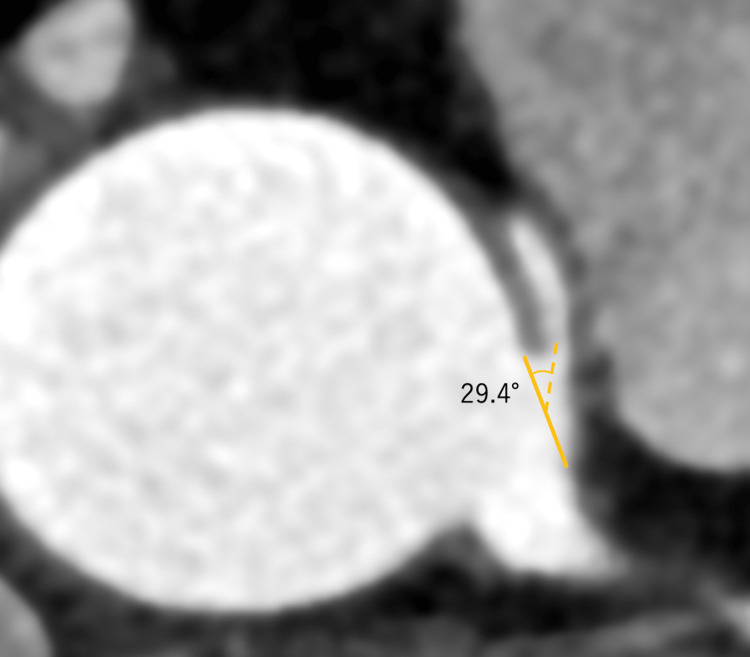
Contrast CT horizontal section at the level of the right coronary bifurcation The solid line is the ostium line, and the dashed line is the line drawn from the midpoint of the ostium line to a point 5 mm along the center of the coronary artery origin. The angle of separation is 29.4°.
